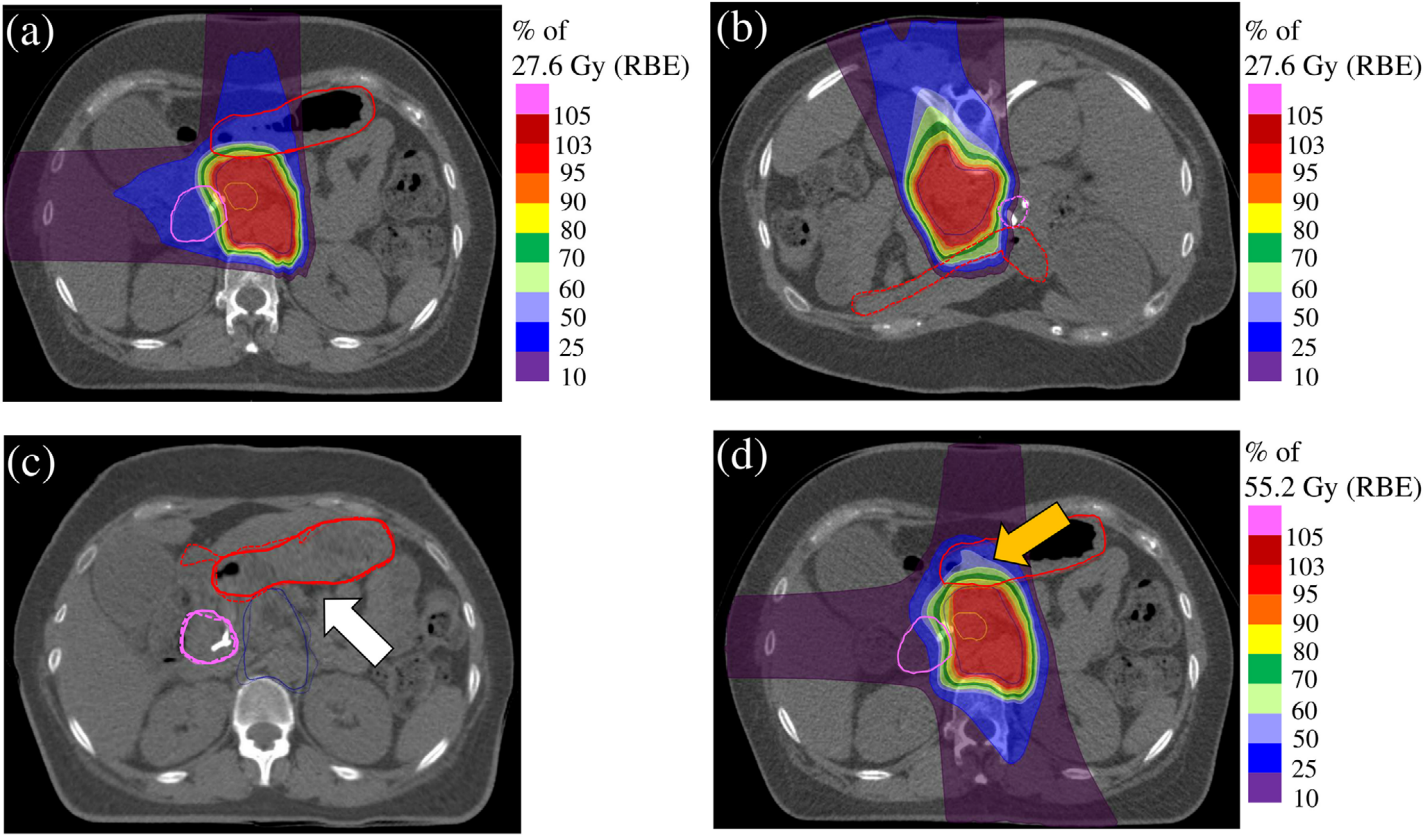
Reference CT image, moving CT image, and accumulative dose distribution formed by DIR. (a) is the supine CT, (b) is the prone CT, (c) is the CT image deformed by DIR, and (d) is an accumulative dose distribution. The CT image was deformed to match the ROI of the stomach, as shown by the yellow arrow. There was a broadening of the isodose line in the area indicated by the arrow in the accumulative dose distribution. CT , computed tomography; CTV, clinical target volume; DIR, deformable image registration; RBE, relative biological effectiveness; ROI, region of interest.
